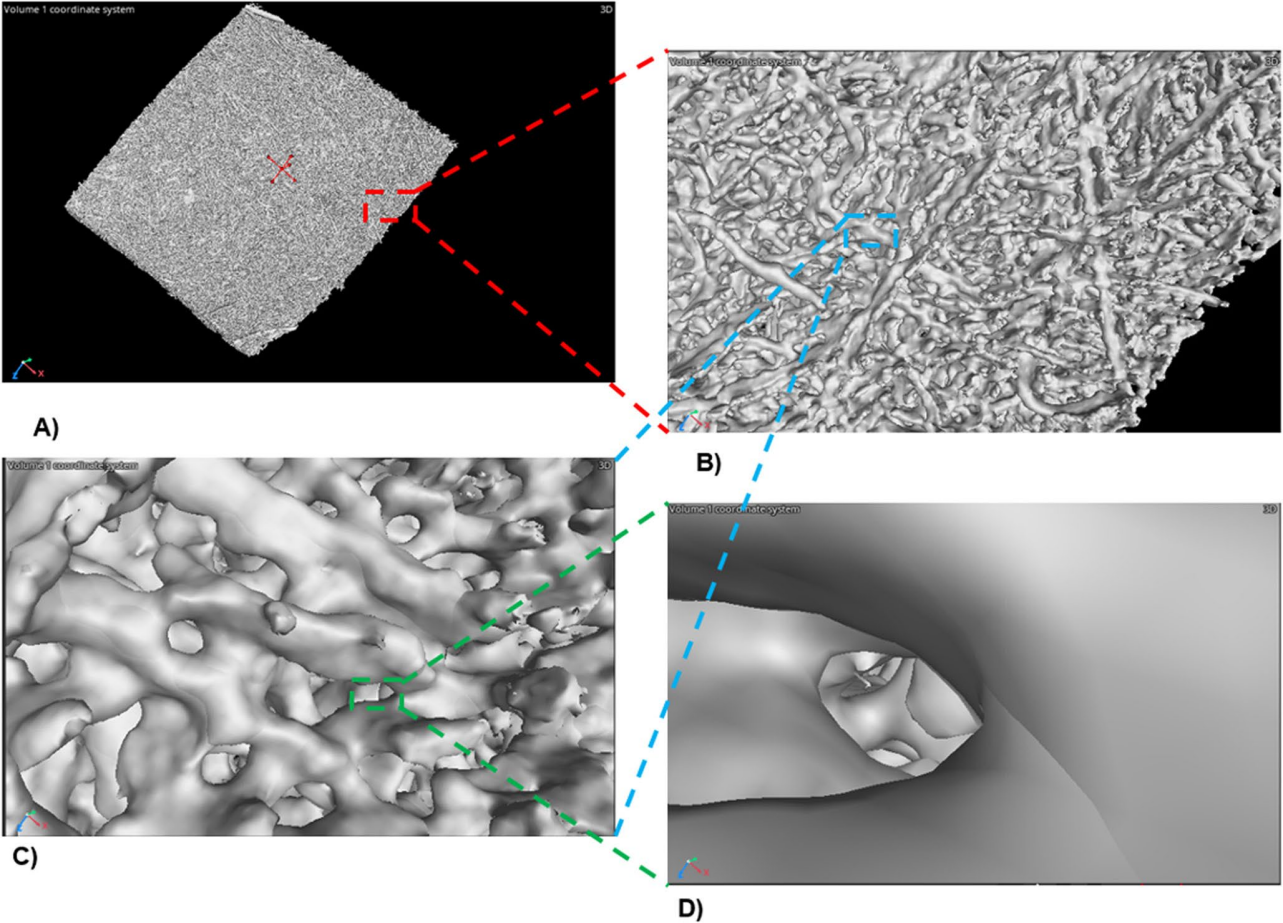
3D volume from X-ray tomography of PCL/PVP and PCL/PVP-ChAgG fibers. Analysis of interconnected pores. Images were developed using software VGSTUDIO MAX (Version 2022.4) (https://www.volumegraphics.com/en/products/vgsm.html).
